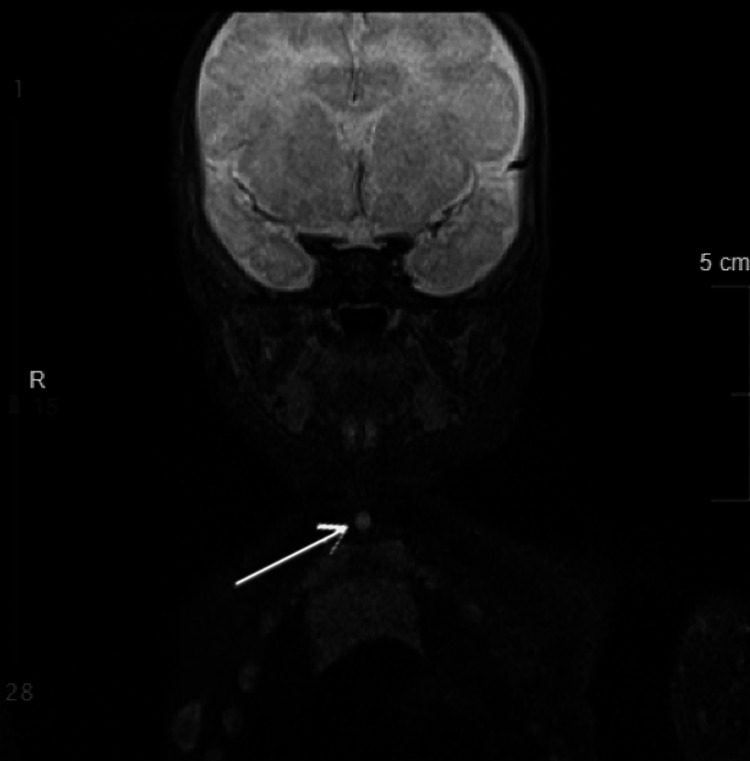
Coronal MRI of the neck demonstrating a midline infrahyoid lesion (arrow) measuring approximately 6 mm, with a small adjacent cystic component (~5 mm), located superficial to the platysma without deep extension, consistent with congenital midline cervical cleft. MRI: magnetic resonance imaging
